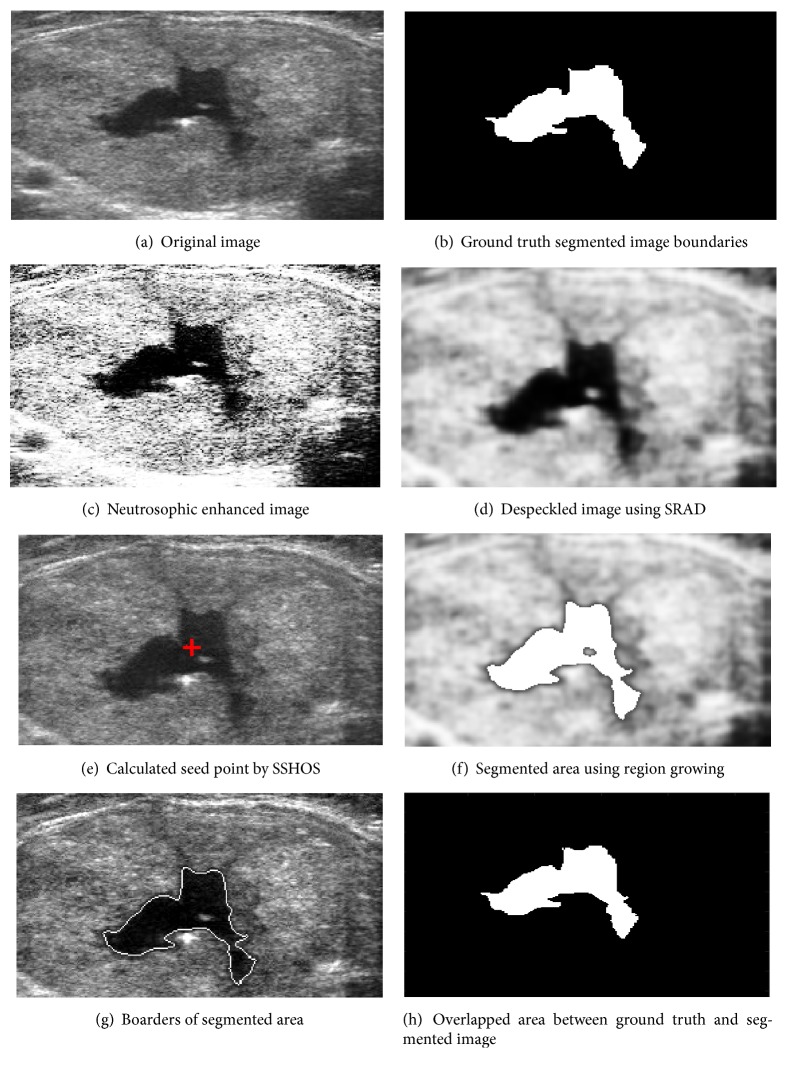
Ultrasound image (image 28B).
